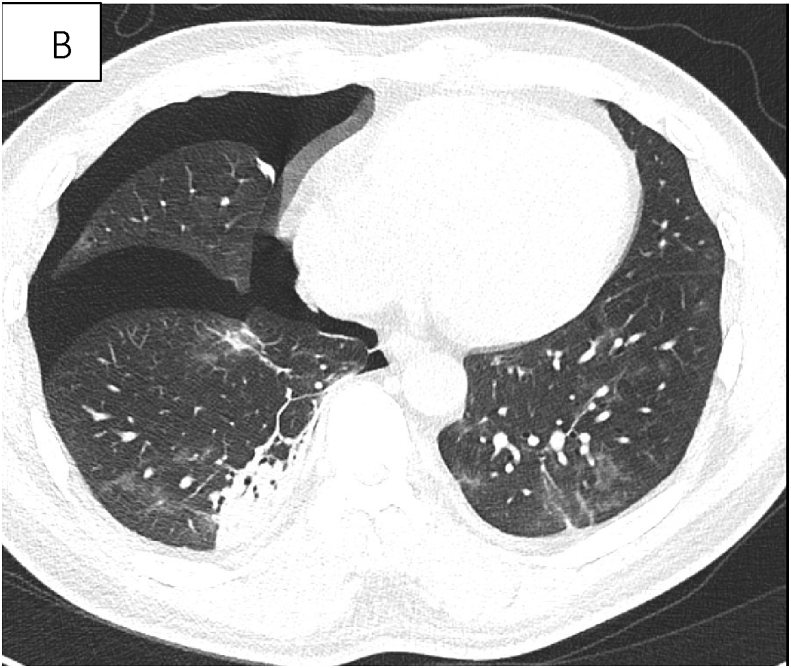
CT chest: Right pneumothorax with multifocal ground glass opacities and segmental consolidation of right lower lobe.
